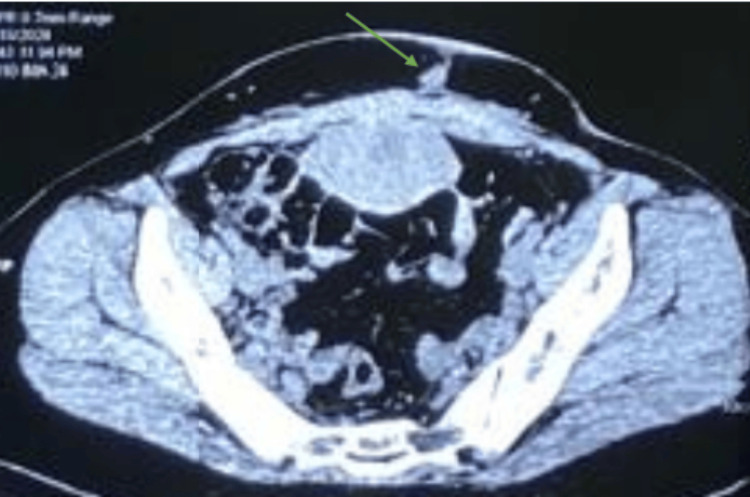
CT abdomen axial section showing a 2.3-cm linear hypodense track (green arrow) extending from the lower anterior abdominal wall to the rectus muscle.
